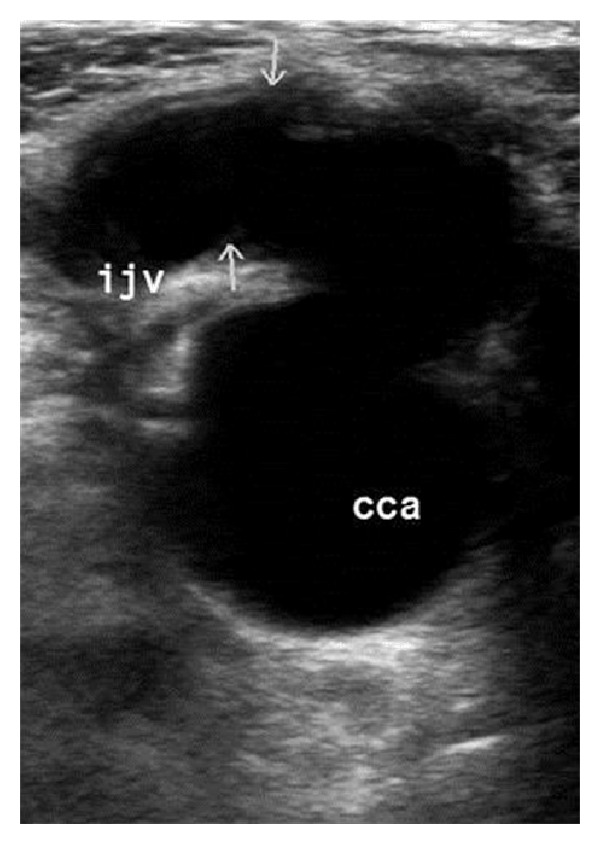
Ultrasound demonstrating fistula connecting right CCA to the right IJV.
